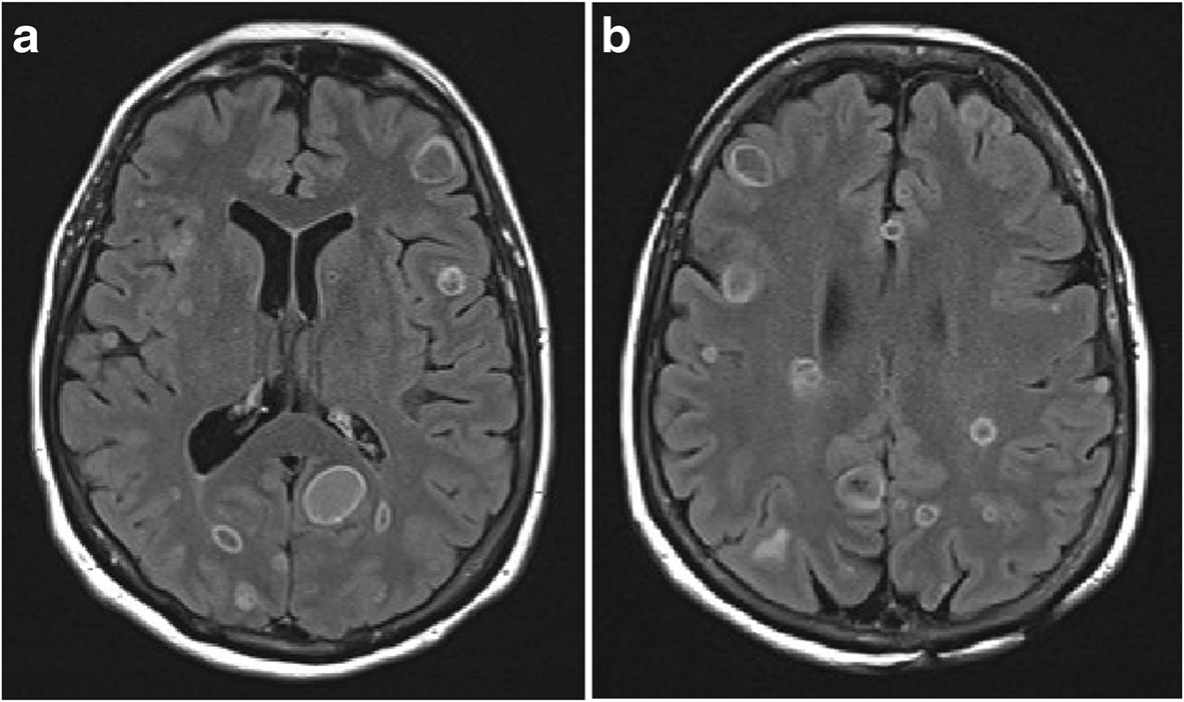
a, b Magnetic resonance imaging of the brain demonstrated multiple bilateral intracranial cystic lesions within the subarachnoid space and parenchyma. Differing signal intensities and fluids levels present on these cystic structures initially suggested neurocysticercosis in multiple stages of evolution
